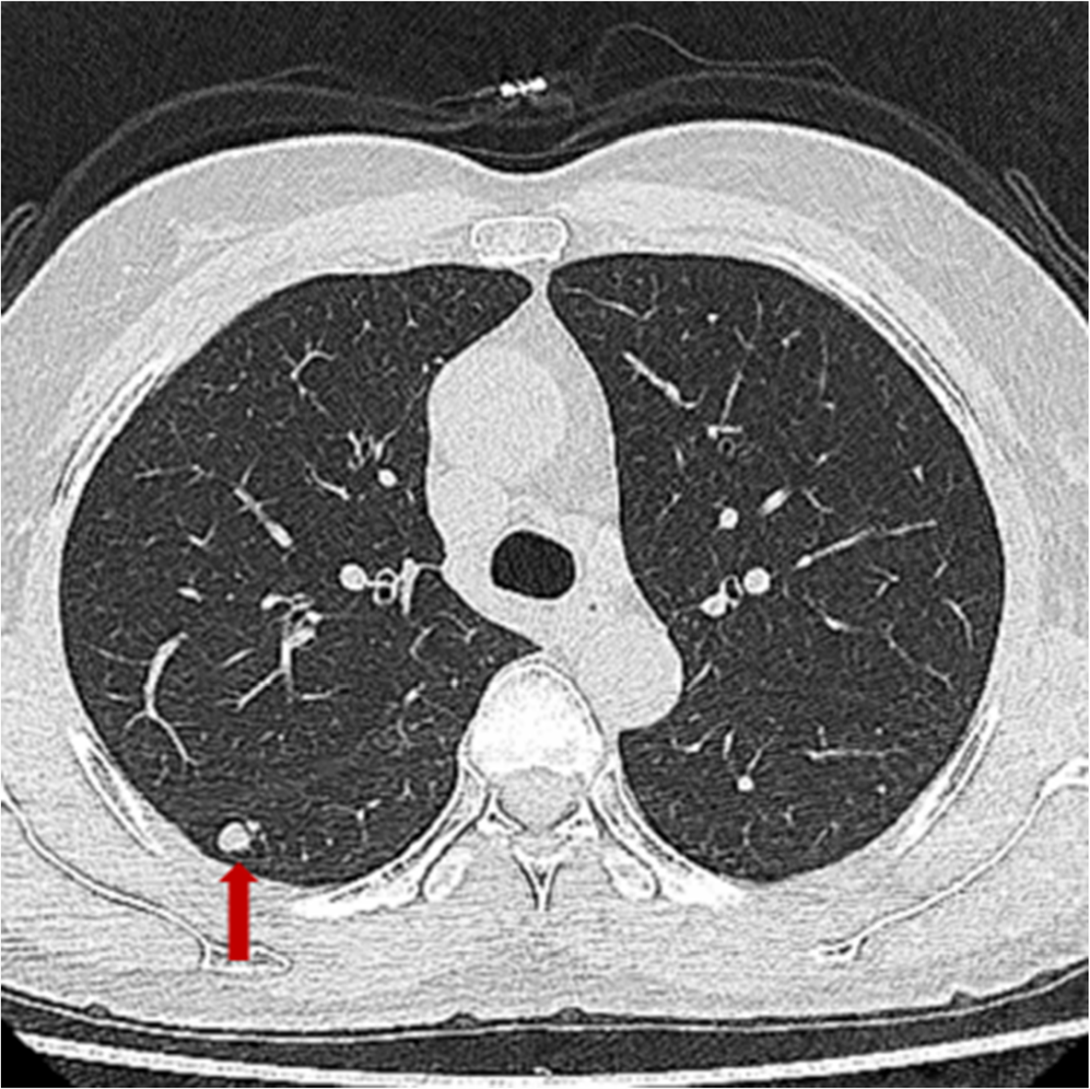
Chest computed tomographic image reveals a well-demarcated, round, solid nodule in the right lung (red arrow)
